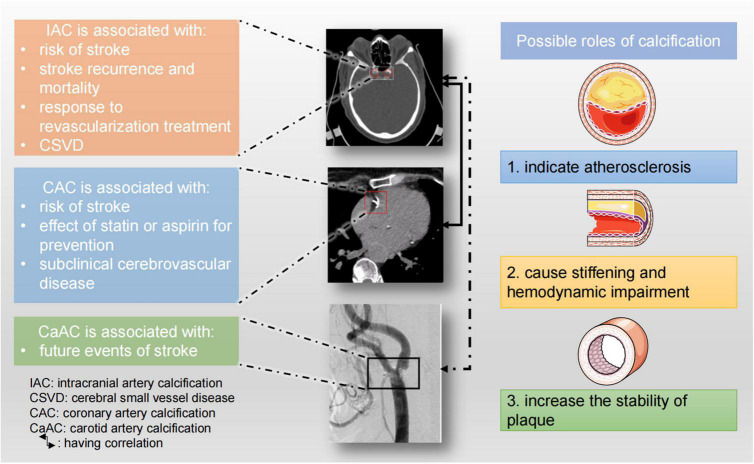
Clinical implications of large artery calcifications and possible roles of vascular calcification. The CT image of IAC was from Rahmani et al. (2022). The CT angiogram of carotid artery calcification was reused with permission of American Society of Neuroradiology, from Tsutsumi et al. (2008); permission conveyed through Copyright Clearance Center, Inc.
